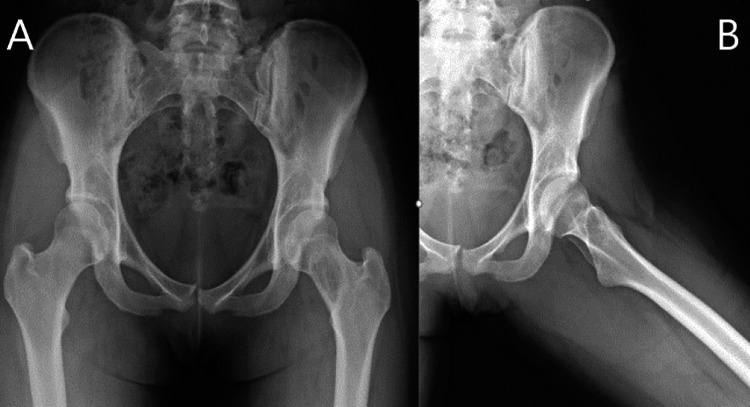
Radiographic anteroposterior (A) and frog-leg lateral (B) views of the hip from initial presentation revealed lesion within the left femoral neck
